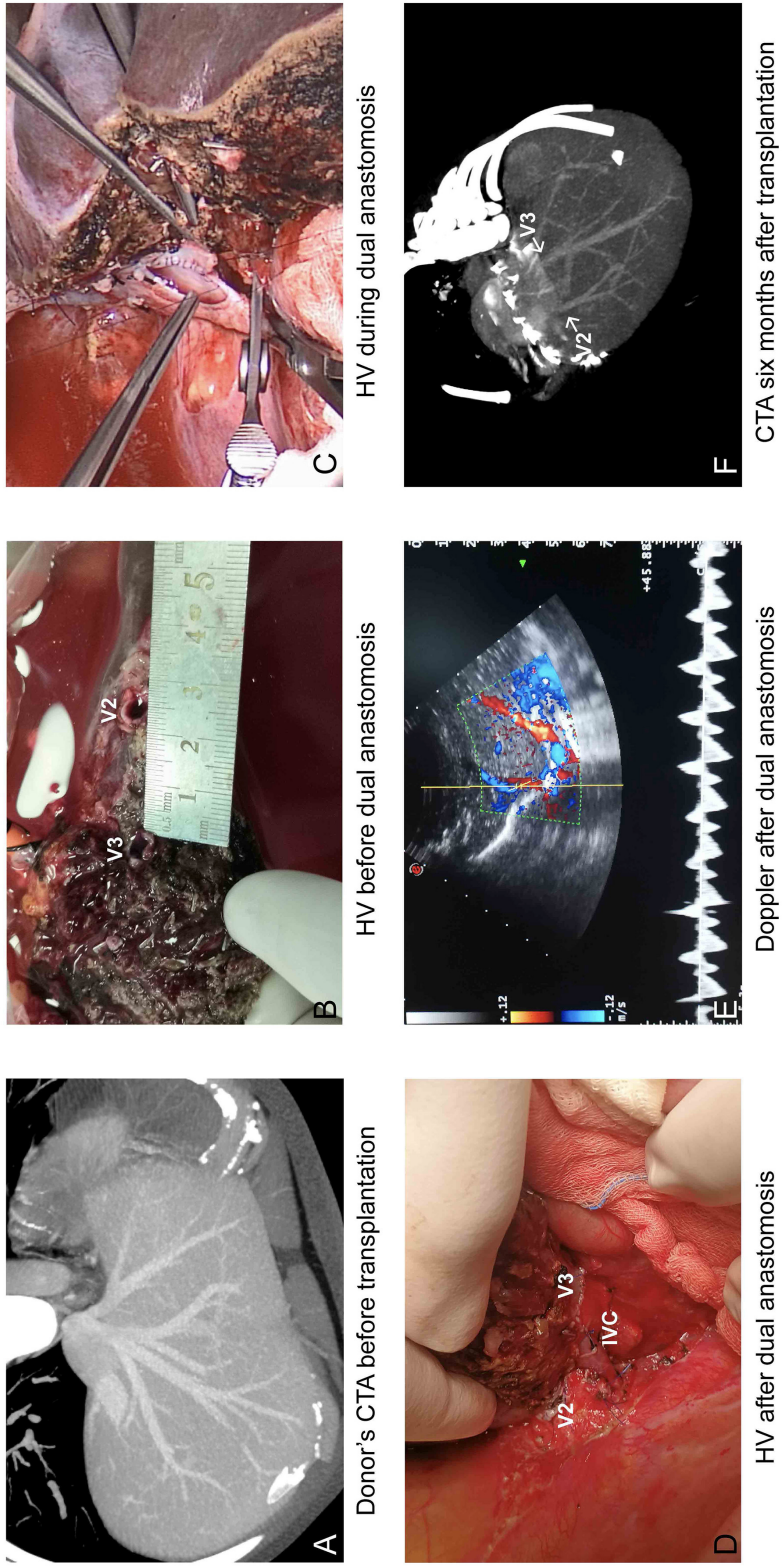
Operational results of modified dual hepatic vein anastomosis. (A) Donor's CTA before transplantation and (B) during transplantation showed two separated orifices in cut surface; (C) V2 was anastomosed to the common trunk of MHV and LHV, and V3 was anastomosed to longitudinal incision orifice in IVC. (D) HV after dual anastomosis. (E) Venous outflow was satisfactory on Doppler. (F) CTA at 6 months after liver transplantation.
